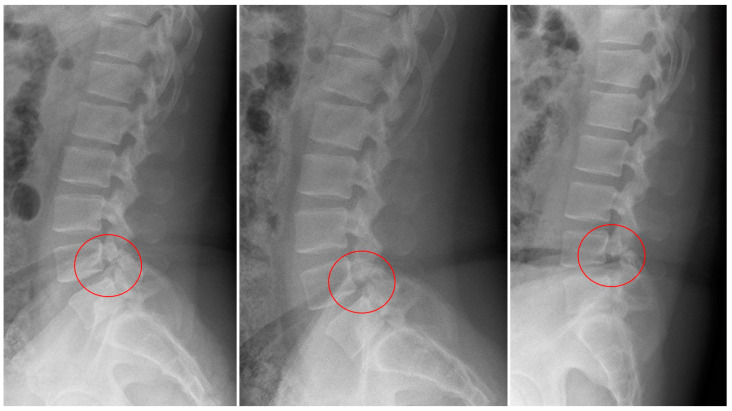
L-spine lateral radiography before surgery shows spondylolisthesis at the L4/L5 level.
